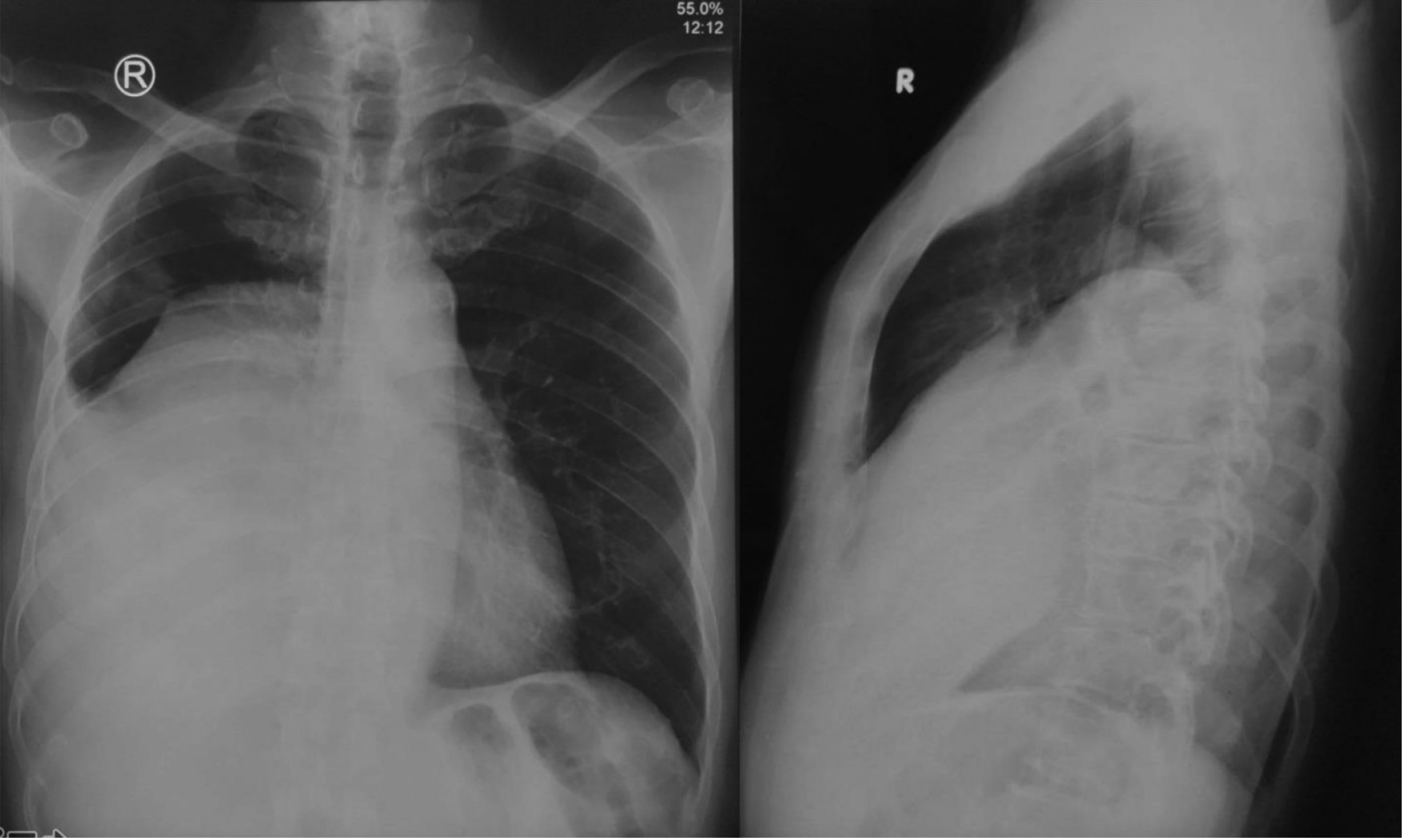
A chest x-ray AP/ Lateral projection showed large opacity with a defined border and regular margin in the right hemithorax covering the right hemidiaphragm and right anterior and posterior costophrenic angle, resembling a pleural effusion.
